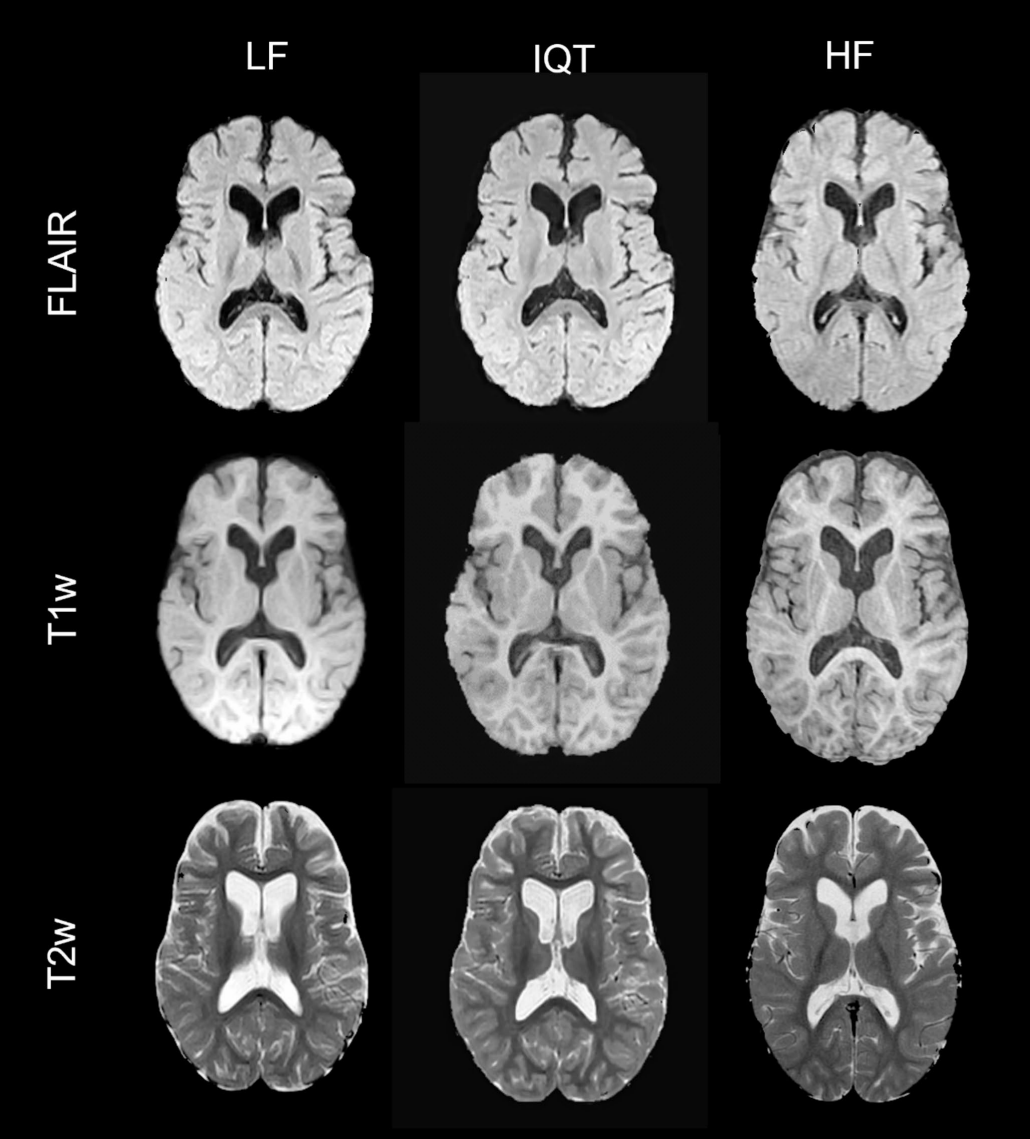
Representative LF, IQT-enhanced and HF axial images for a 12-year-old patient with lesion-negative brain MRI and generalised seizures. On visual inspection, there is an improvement of the grey/white matter differentiation visible in T2w and T1w images
